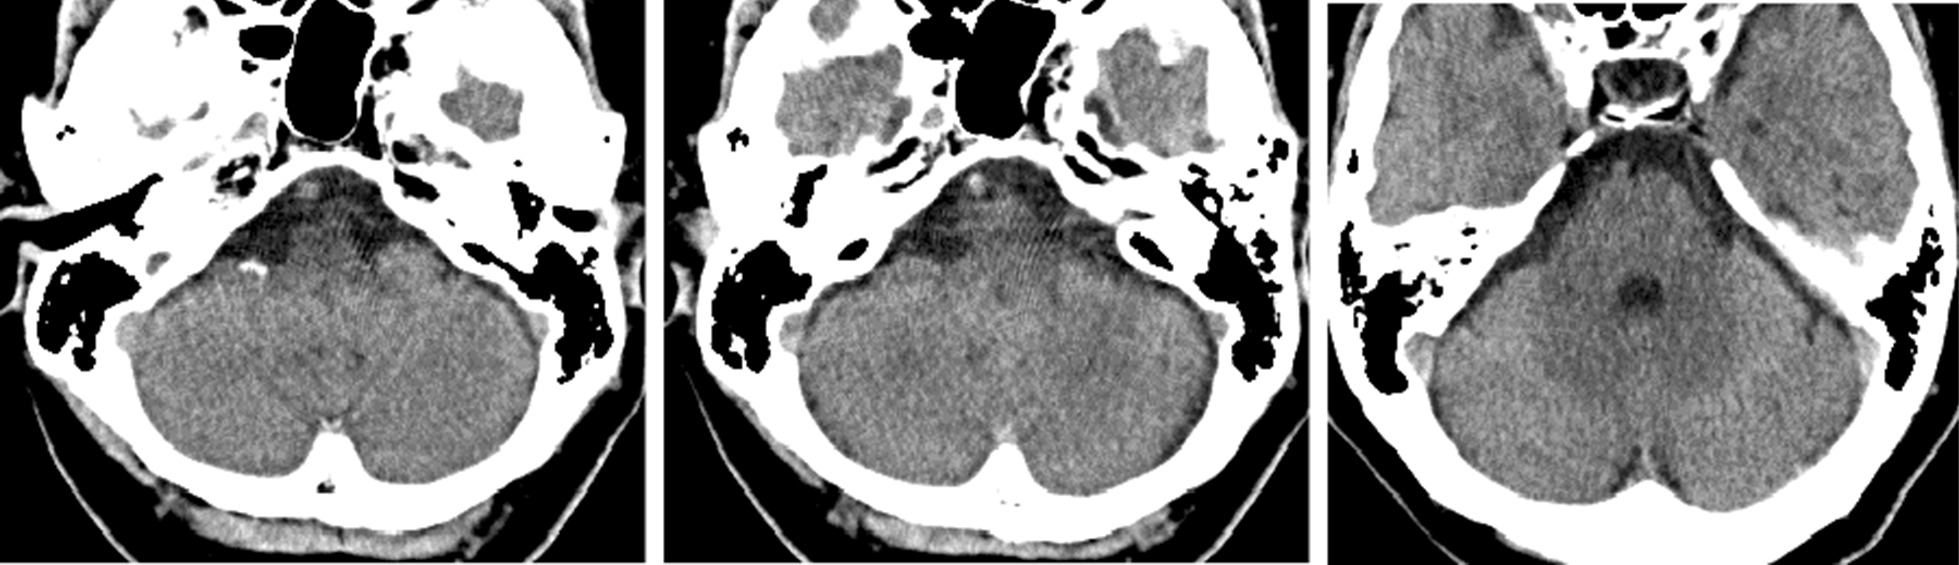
Brain computed tomography showed no new lesions in the area of the cerebellar hemispheres and brainstem
